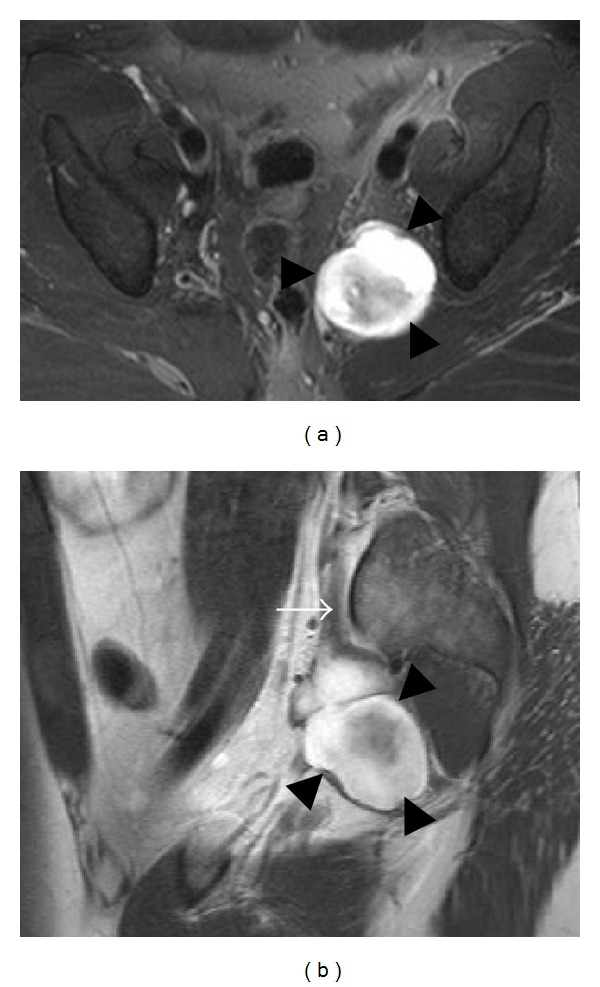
Surgically proven neurofibroma of the left sciatic nerve in a 33-year-old patient. Axial T2-weighted fat-saturated (a) and sagittal T1-weighted postcontrast (b) images show a lobulated enhancing mass (arrowheads) with isointense T1 (not shown) and high T2 signal originating from the left sciatic nerve. Proximally, the left S1 nerve root is thickened (white arrow). Note the target appearance of the neurofibroma.
